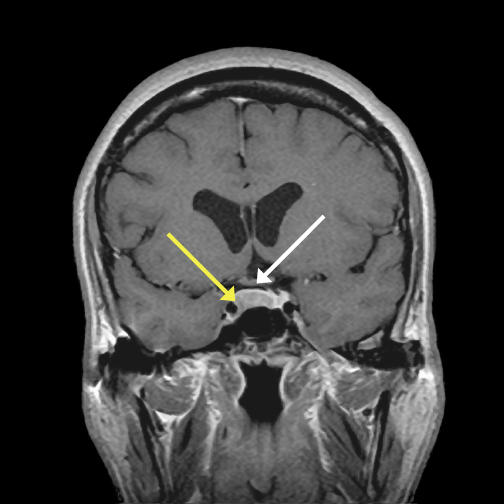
MRI Studies of the Patient's Pituitary Gland MRI of the pituitary gland showing a non-enhancing lesion occupying the bulk of the pituitary fossa (yellow arrow). The remaining normal pituitary is pushed to the left, as is the pituitary stalk. The optic chiasm can be seen just superior to the pituitary gland (white arrow).
